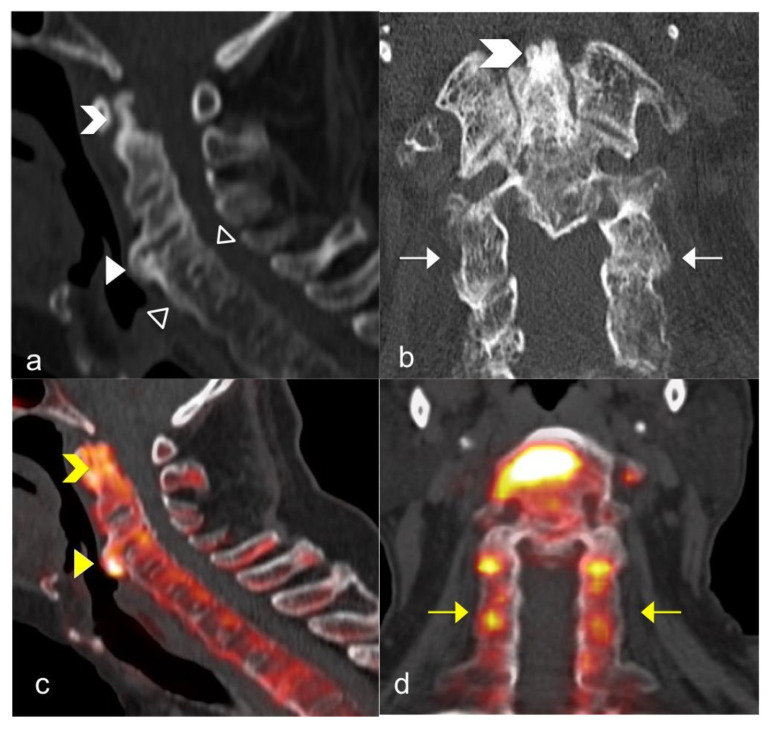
A 66-year-old man with axial PsA. The CT image on the sagittal plane (a) shows extensive new bone formation with bridging-syndesmophytes along the anterior and posterior corner (empty white arrowheads) also with bridging-osteophytes between C3-C4 level (white arrowhead); CT images on the coronal plane (b) show extensive structural damage with bony erosion and sclerosis of dens (white chevron) also with multilevel ankylosis of facet joints (white arrows). Sodium 18F-Fluoride PET-CT of the cervical spine on the sagittal (c) and coronal plane (d) shows an increase in tracer uptake at the dens (yellow chevron), at the bridging-osteophytes between C3-C4 level (yellow-arrowhead) and along the facet joints (yellow-arrows).
